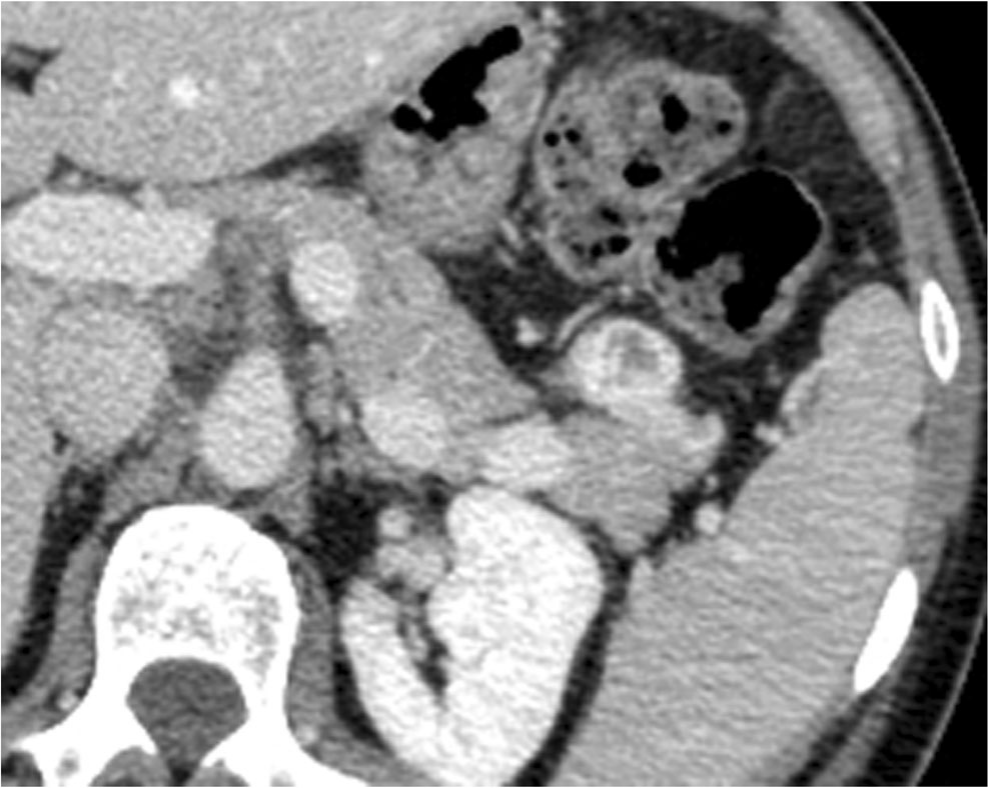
Cystic NEN. CT examination: small cystic exophytic pancreatic tail mass with hypodense central area surrounded by thick hypervascular rim
